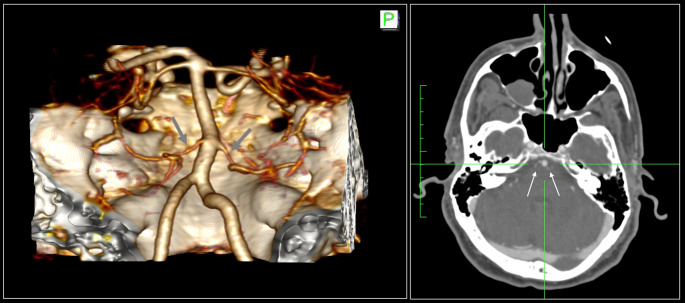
Computed tomography angiography (CTA) with 0.8 mm slice thickness. Three-dimensional volume and axial reconstructions of bilateral posteroinferior cerebellar artery (PICA) originating from the basilar artery (BA)
